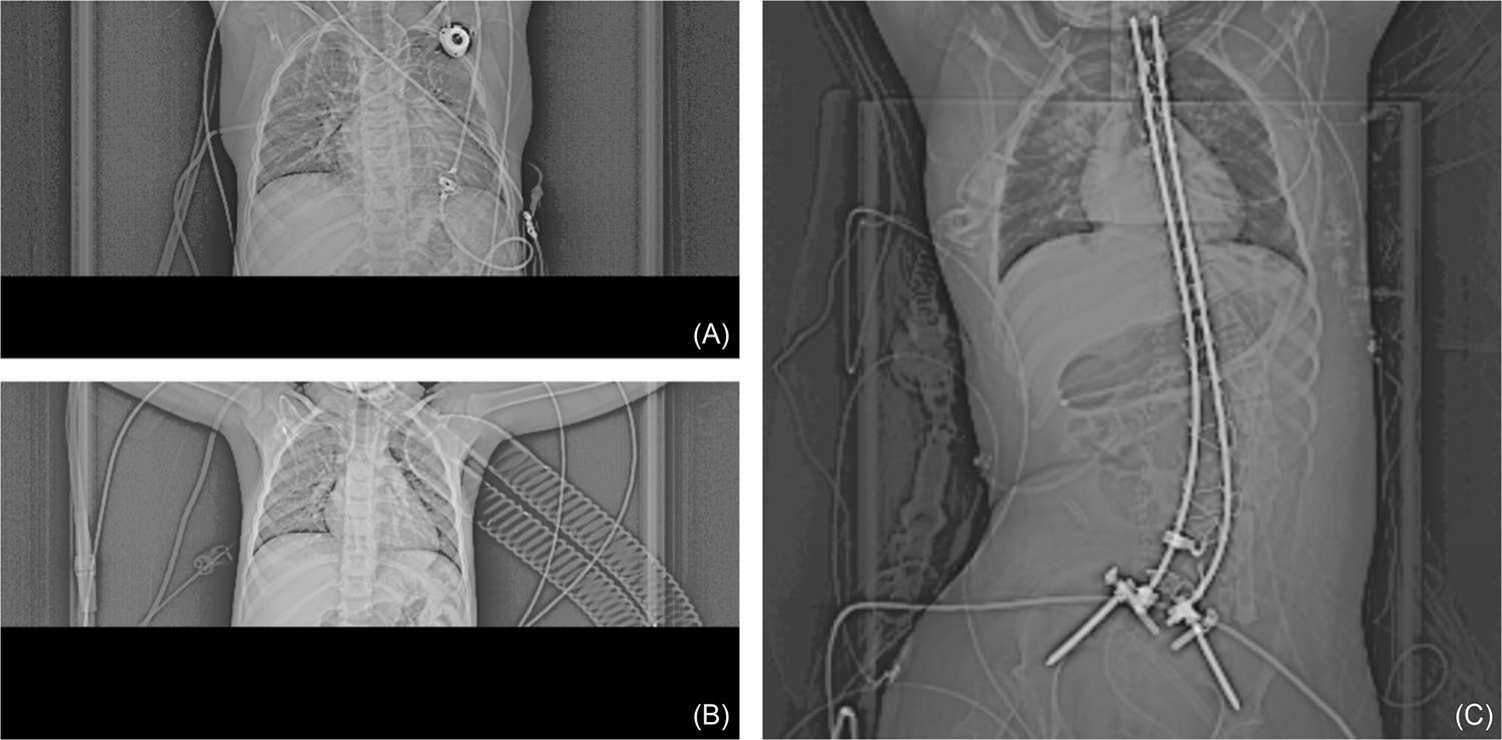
Preprocessed CT scout views for two patients. (A) The CT scout view for a CT thorax examination of a male patient (5.3 years) (B) The CT scout view for a CT thorax examination of a male patient (2.8 years) (C) The CT scout view of a CT abdomen examination of a female patient (19.5 years).
